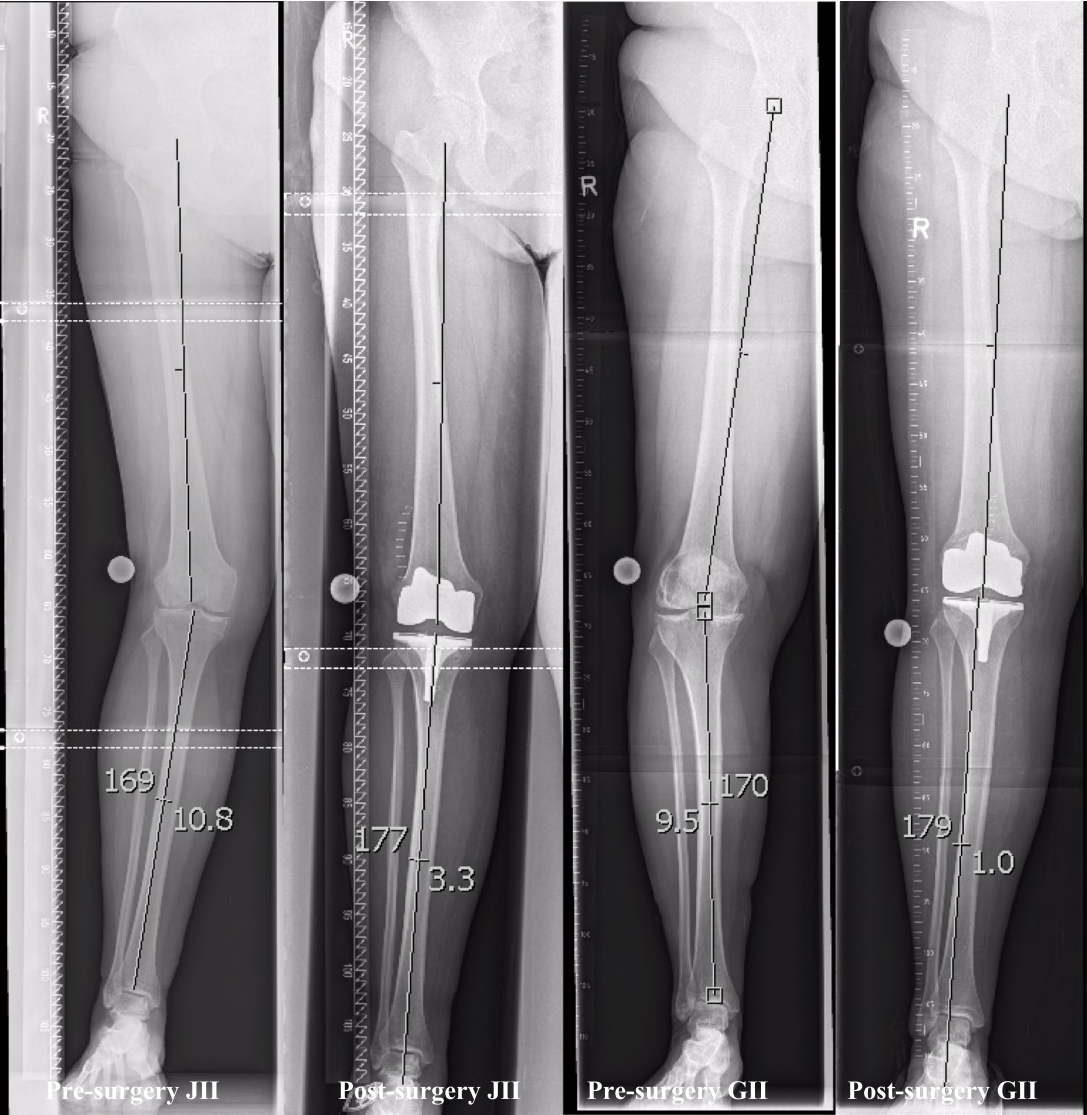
Figures of angle measuring pre- and postoperative with JII and GII arthroplasty; The fixed points were the hip joint center of rotation, the distal femoral center, the proximal tibial center, and the distal tibial center. Valgus angles deviate lateral at the tibial site, varus angles to the medial site;.Measuring depended strongly on the quality of the radiograph;
